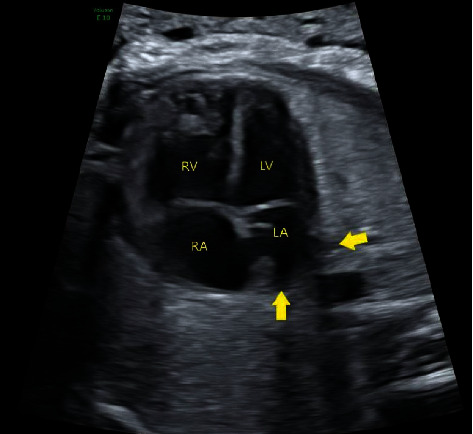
Standard 2D greyscale ultrasound image of the 4-chamber view of the fetal heart with pulmonary vein entry into the left atrium (LA) indicated by yellow arrows (legend: LA = left atrium, RA = right atrium, LV = left ventricle, and RV = right ventricle.).
